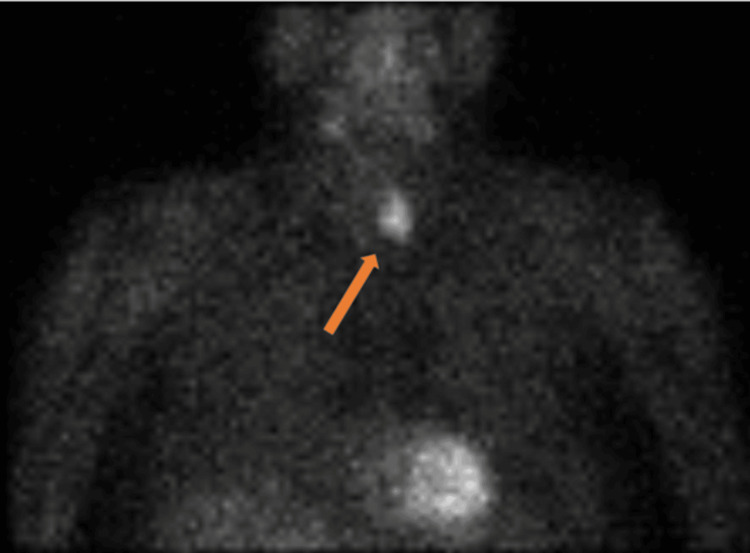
Nuclear medicine parathyroid SPECT – coronal uptake image Increased uptake within the soft tissue mass is evident (orange arrow). SPECT - single photon emission computed tomography
